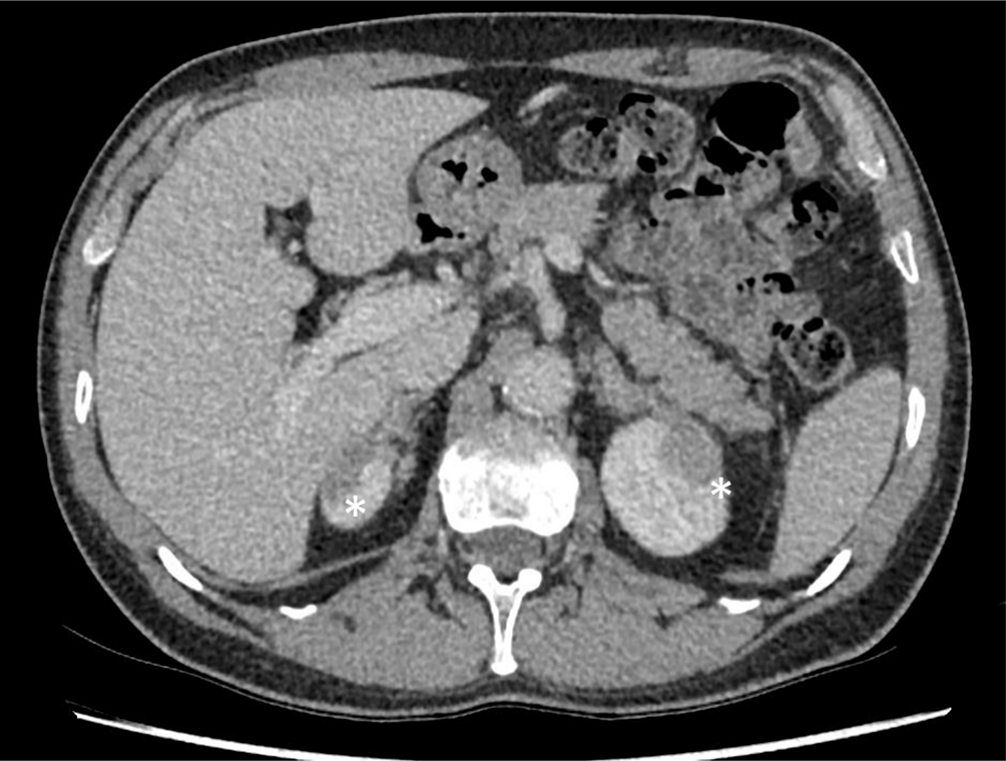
Axial and contrast-enhanced CT image shows bilateral symmetrical well-defined
